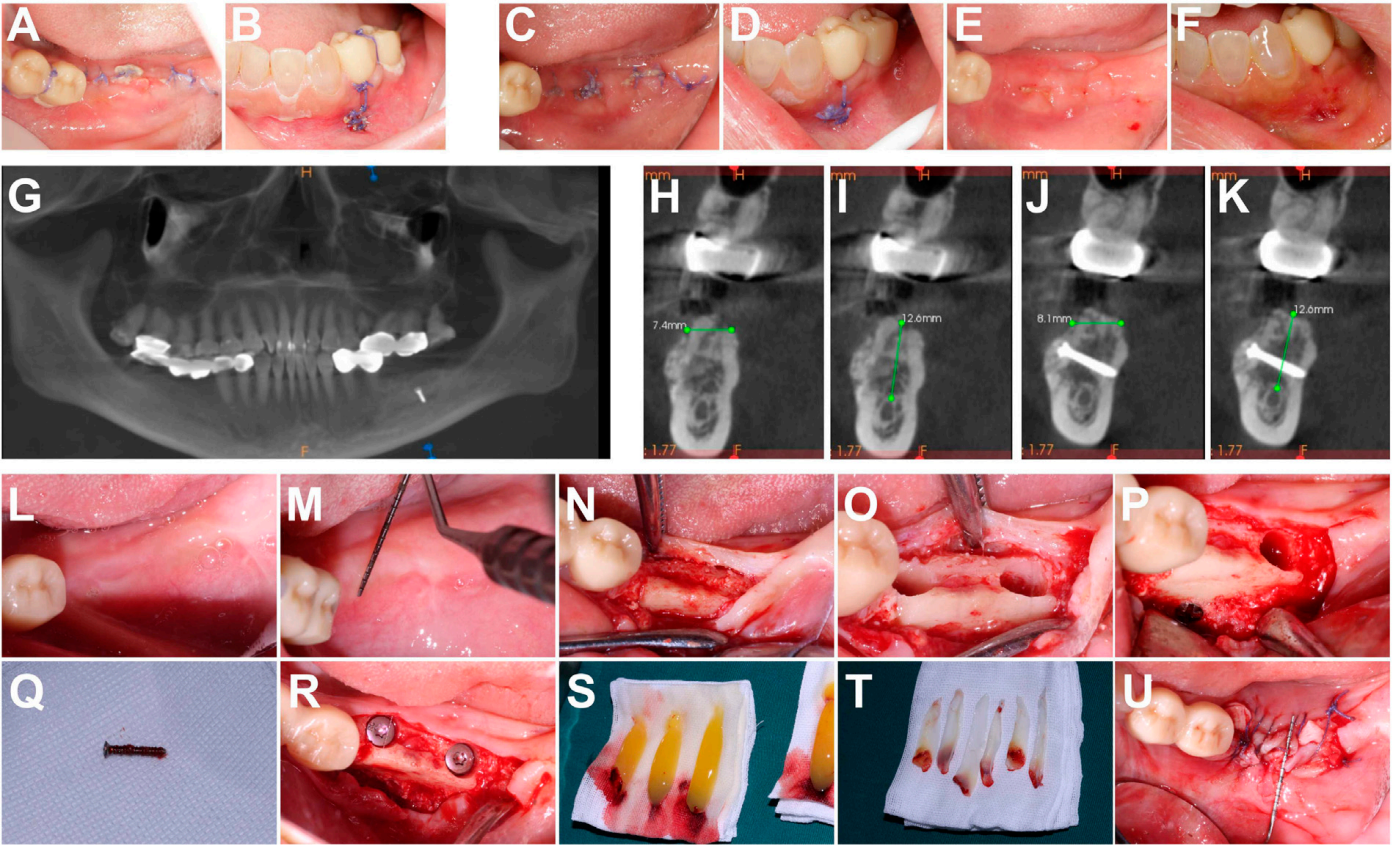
(A, B) Postoperative intraoral view on the seventh day. (C–F) Postoperative intraoral view on the sixteenth day (after suture removal). (C, D) Before suture removal. (E, F) After suture removal. (G–K) CBCT on the seventh month after the follow-up visit. (H, I) Implant sites #36. (J, K) Implant sites #37. (L–U) Titanium nail removal and implant surgery process.
